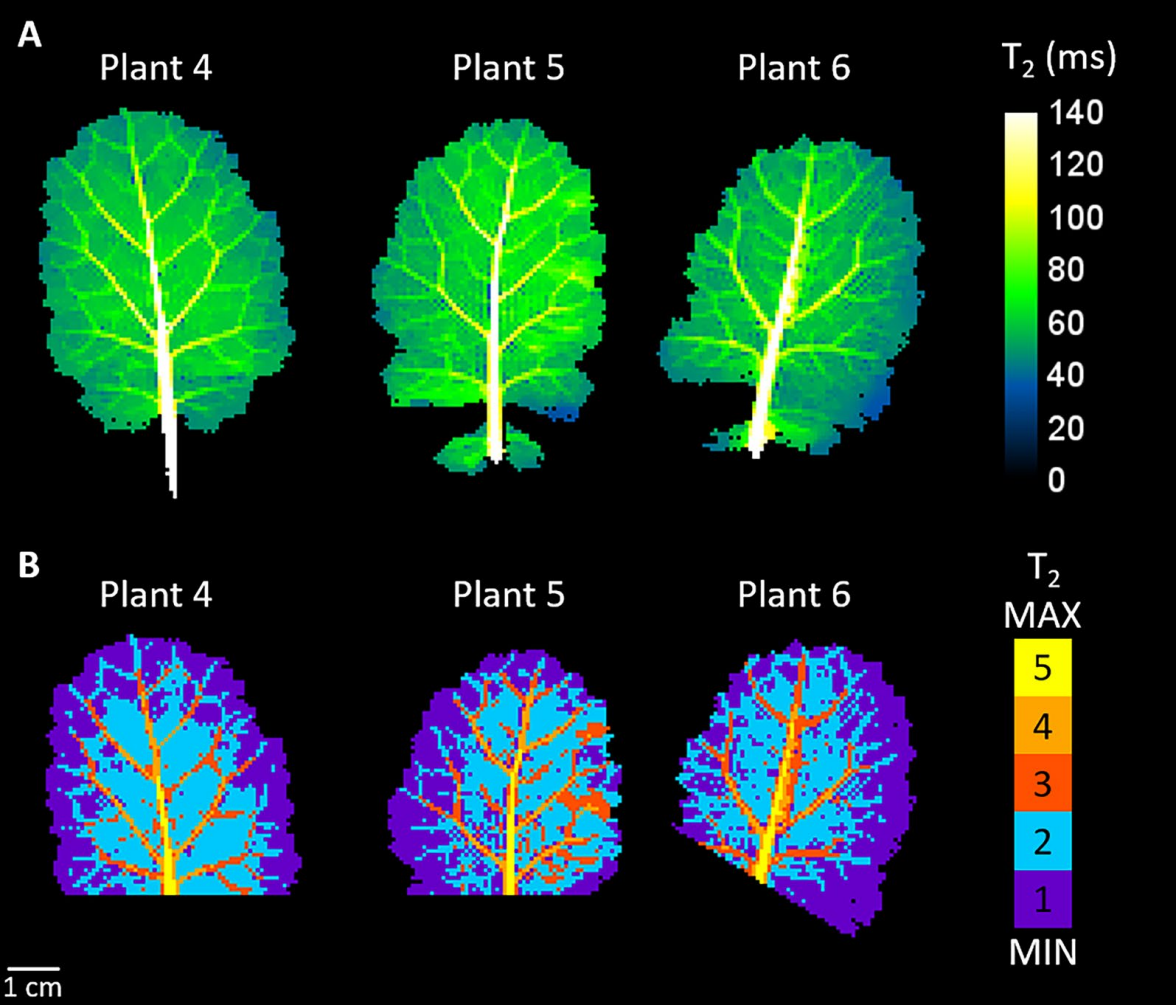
Transverse relaxation (T_2_) maps of 3 mature (leaf rank 3) leaves computed from *in planta*-acquired MRI images (A) and their corresponding classifications (B)
